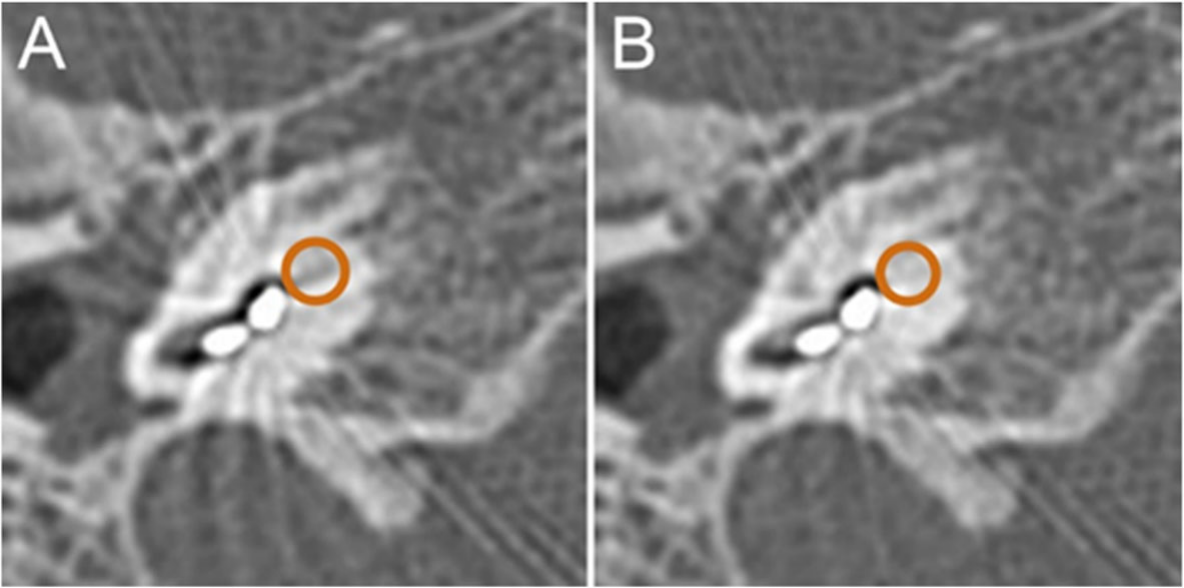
a ROI on a CT image without metal artifact removal. b ROI on a CT image with metal artifacts removed. ROI: regions of interest
